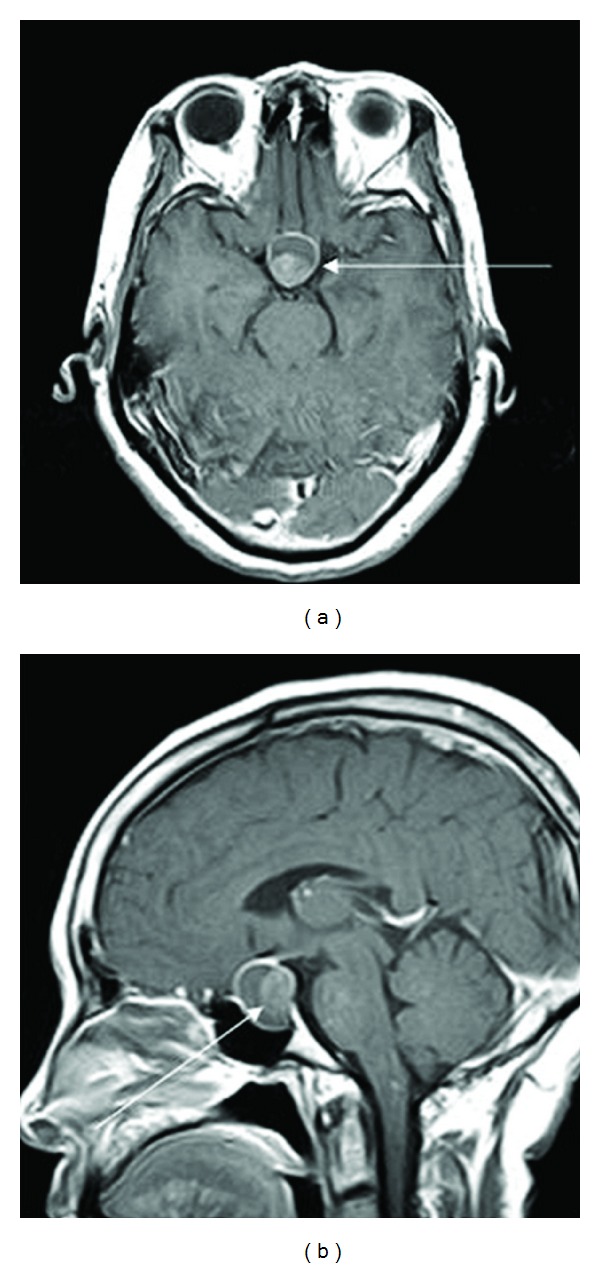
Case 2: enhanced MRI T1-weighted images. Intracystic nodules with hyperintense signal on T1-weighted images in the posterior RCC (arrow).
